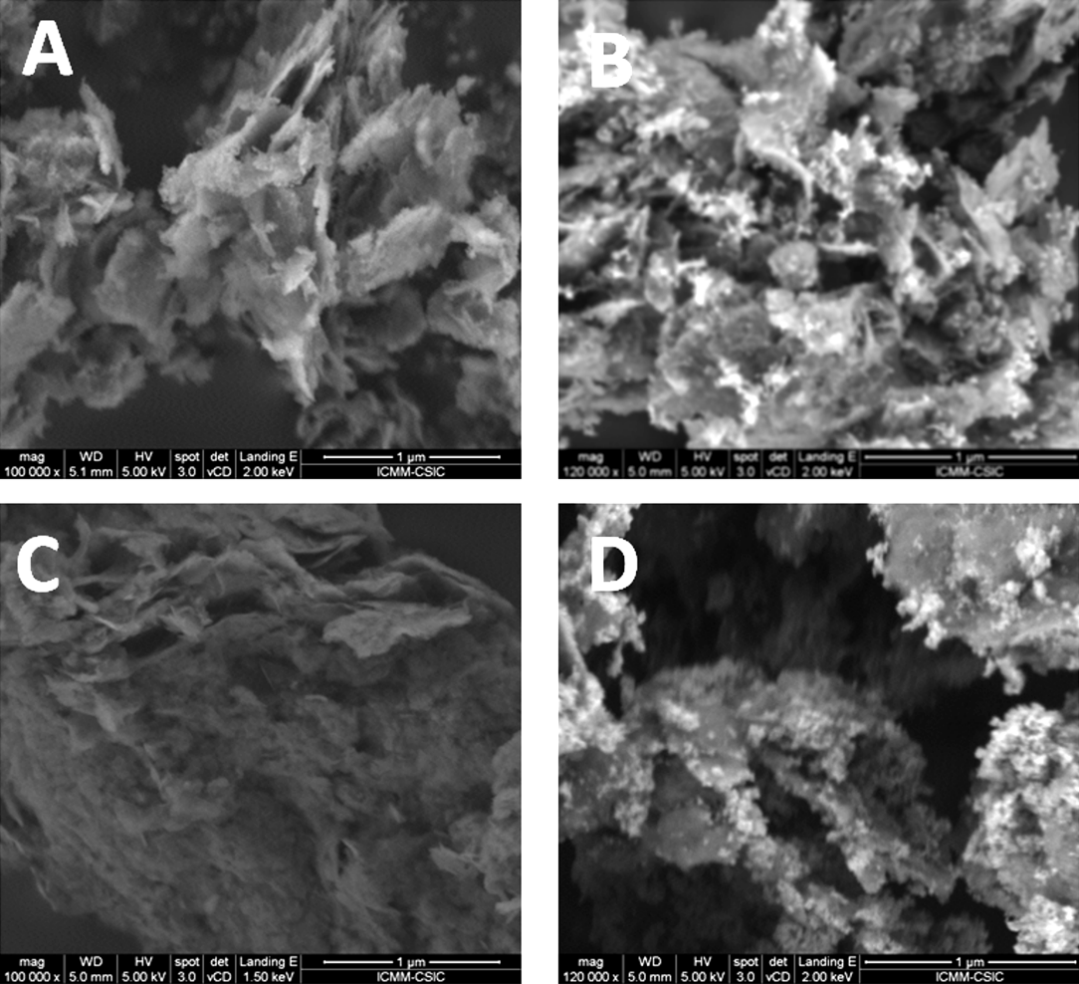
FE-SEM images of the heterostructures: (A) SiO_2_-CLO-CTA, (B) ZnO/SiO_2_-CLO, (C) SiO_2_-TSM-CTA and (D) ZnO/SiO_2_-TSM.
